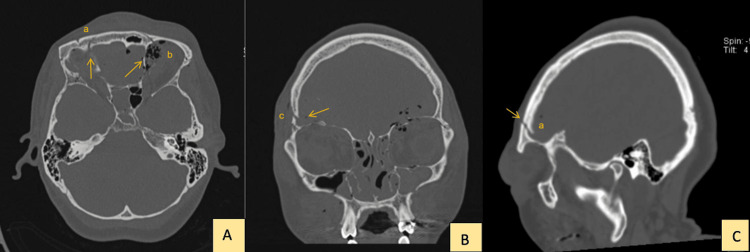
Craniofacial CT (bone window) showing bilateral frontal fractures with right-sided depression and associated orbital injuries. A (axial, non-contrast): Bilateral frontal bone fractures (a) with a depressed right frontal component and adjacent air locules/pneumocephalus (b) (arrows). B (coronal reformat): Comminuted fracture of the right lateral orbital wall extending to the orbital surface of the ipsilateral greater wing of the sphenoid (c) (arrow). C (sagittal reformat): Depressed right frontal fracture involving the orbital roof (a) (arrow).
